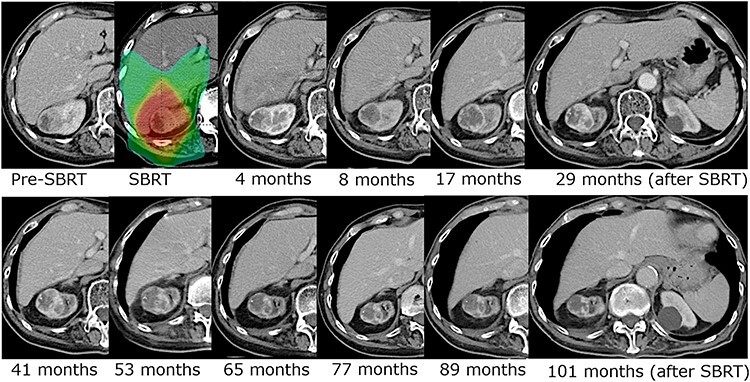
Contrast-enhanced CT images of a long-term follow-up case. Seventy Gy in 10 fractions was prescribed. The volume of the irradiated tumor and enhanced lesion increased slightly in the first four years after SBRT, and then the tumor volume decreased very slowly.
